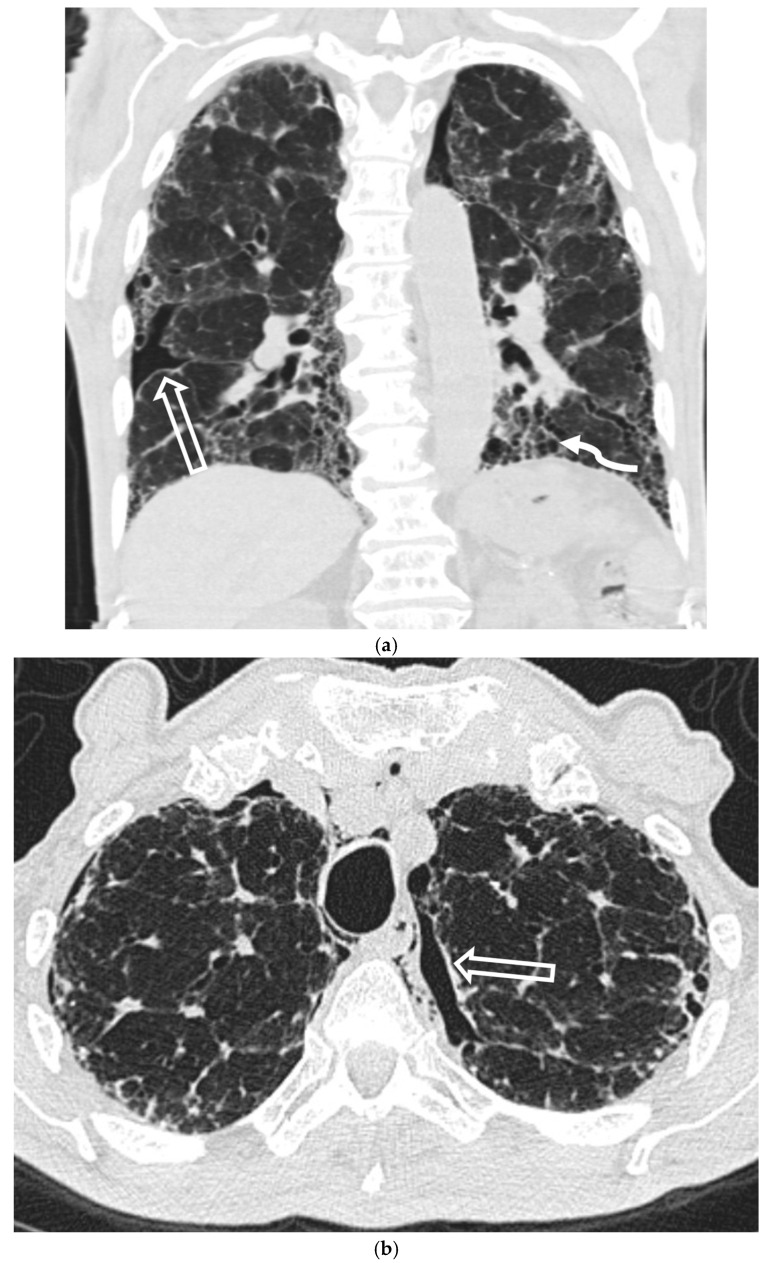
84-year-old male with a history of interstitial lung disease presents with dyspnea. Coronal and axial CT images in lung window (a,b) show peripheral basilar-predominant reticulation (curved white arrow), mild ground-glass opacity, architectural distortion, traction bronchiectasis, and honeycombing characteristic of idiopathic pulmonary fibrosis, along with small bilateral pneumothoraces (open white arrows) and small pneumomediastinum.
